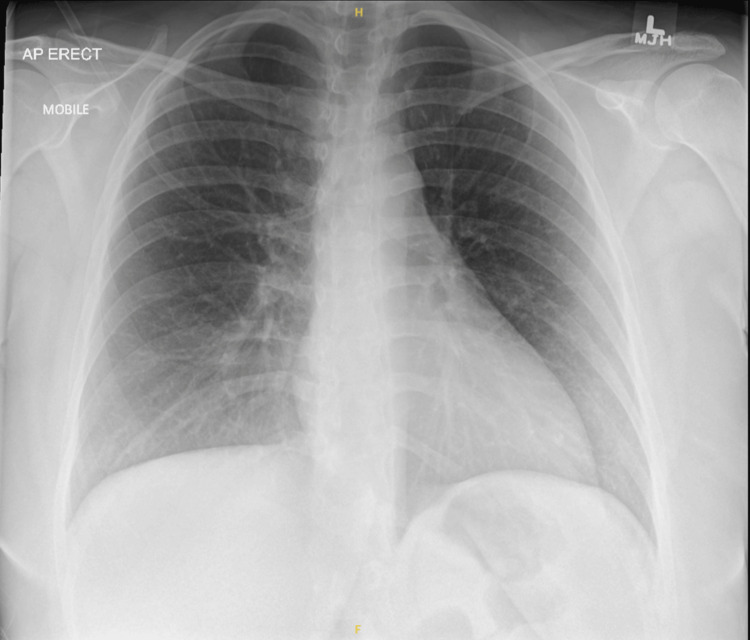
Normal chest X-ray of the patient
